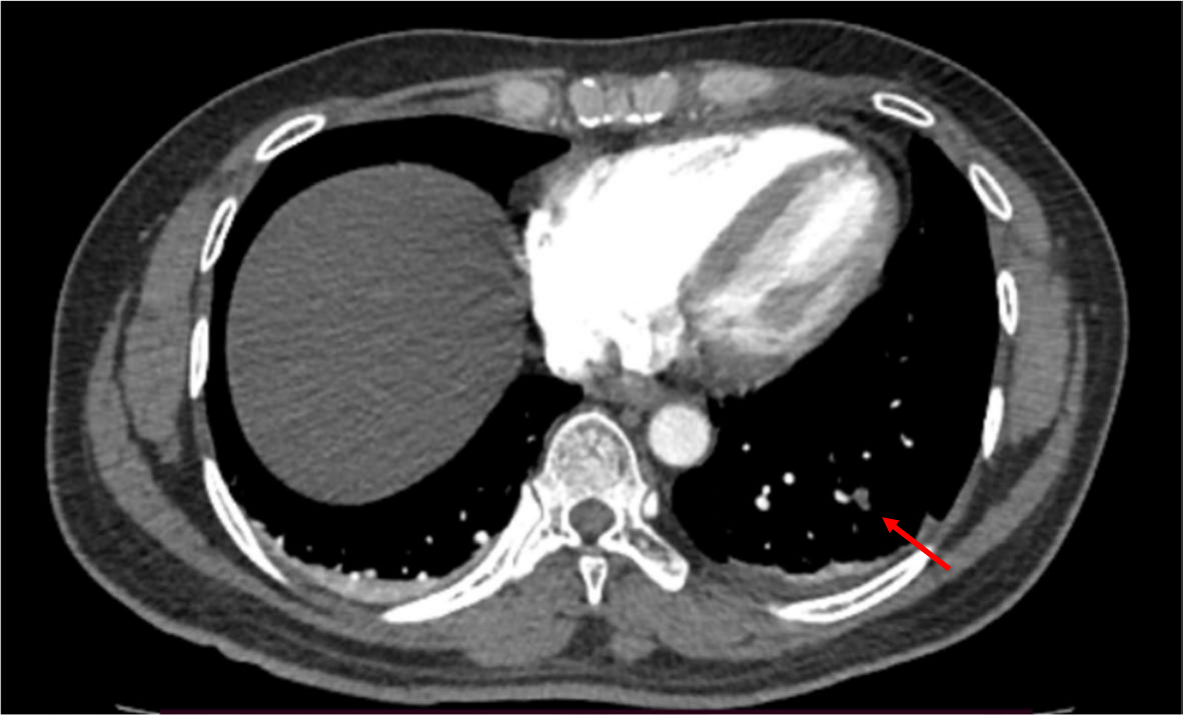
A CT pulmonary angiogram revealed a pulmonary embolism in the left posterior segment pulmonary artery
